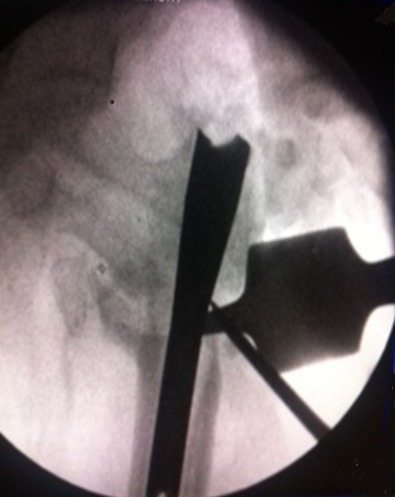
Intra-operative antero-posterior radiograph of materiel ablation
